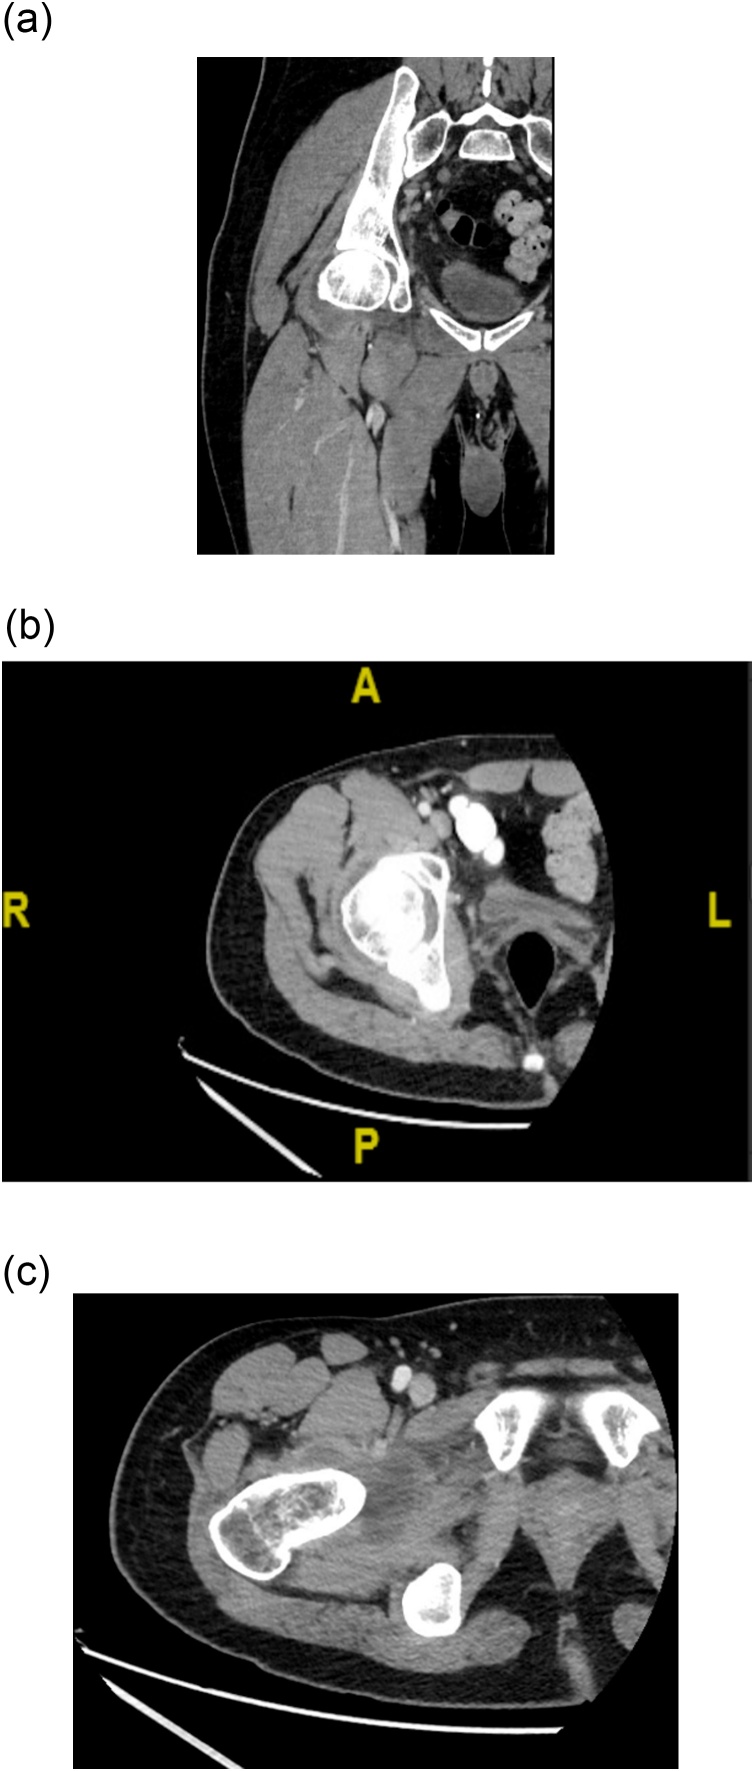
Representative axial and coronal computed tomography (CT) images of the right lower extremity with contrast depicting moderate right hip joint effusion.
